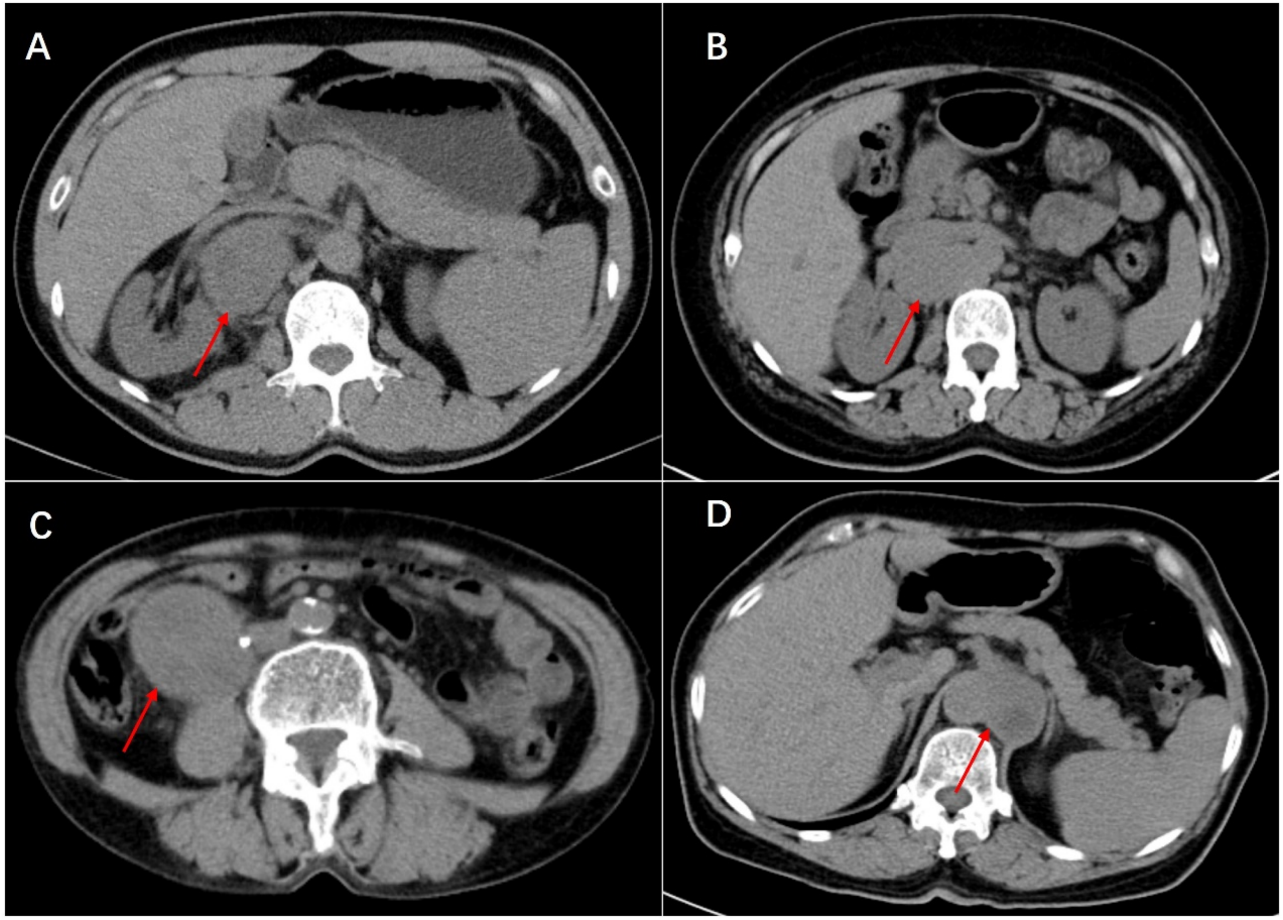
Plain scan of Castleman disease (A), lymphoma (B), leiomyosarcoma (C) and paraganglioma (D) in enhanced CT scan. Multiple enlarged small lymph nodes can be seen around lesion of Castleman disease and lymphoma. In the image, the leiomyosarcoma can be seen invading the right ureter, resulting in secondary hydronephrosis (not shown in the image), which was managed with a ureteral stent placement before surgery. In particular, splenomegaly can be seen in Castleman disease. They are all cases that have been definitely diagnosed by postoperative pathology.
